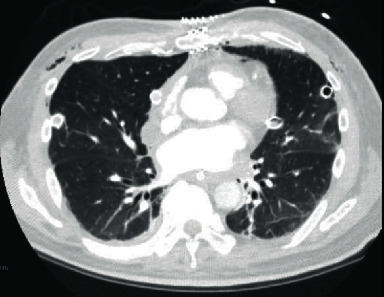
Lung CT scan after bilateral lung transplant.
